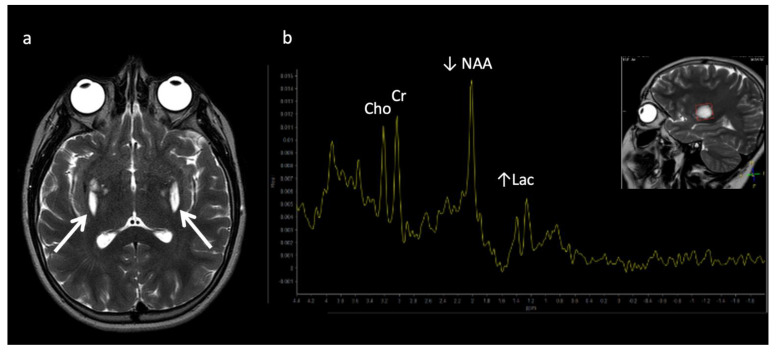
Leigh syndrome in a 10-year-old male with progressive right upper extremity weakness and left leg pain. (a) Axial T2WI shows T2 hyperintense necrotic lesions in the lentiform nuclei (arrows). (b) SV-MRS (short TE) over the basal ganglia shows increased Lac at 1.3 ppm consistent with anaerobic metabolism (indicating active on chronic disease, given the MRI appearance) and reduced NAA.
